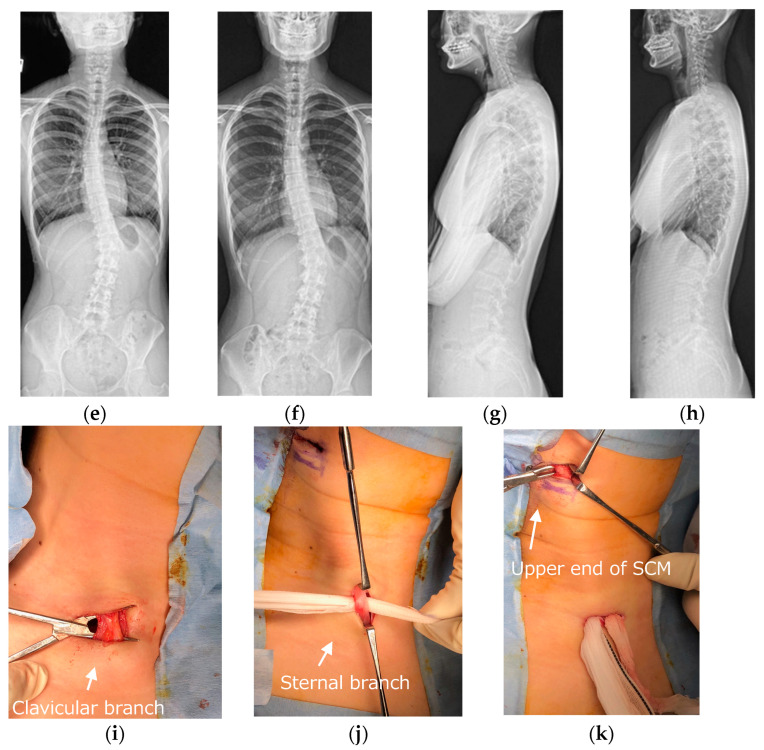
Sixteen-year-old female was treated with the bipolar release of the sternocleidomastoid. Pre- (a,b,e,g) and postoperative (c,d,f,h) appearance, radiographs, and intraoperative photographs. The head was markedly tilted to the right side, and the neck was shifted to the left side preoperatively. Right lower ends of the sternal branch and clavicular branch of the sternocleidomastoid (SCM) were released at one incision (i,j). The upper end of the SCM was released at the other incision (k). There were good improvements in appearance and postoperative X-rays after the bipolar release of SCM.
